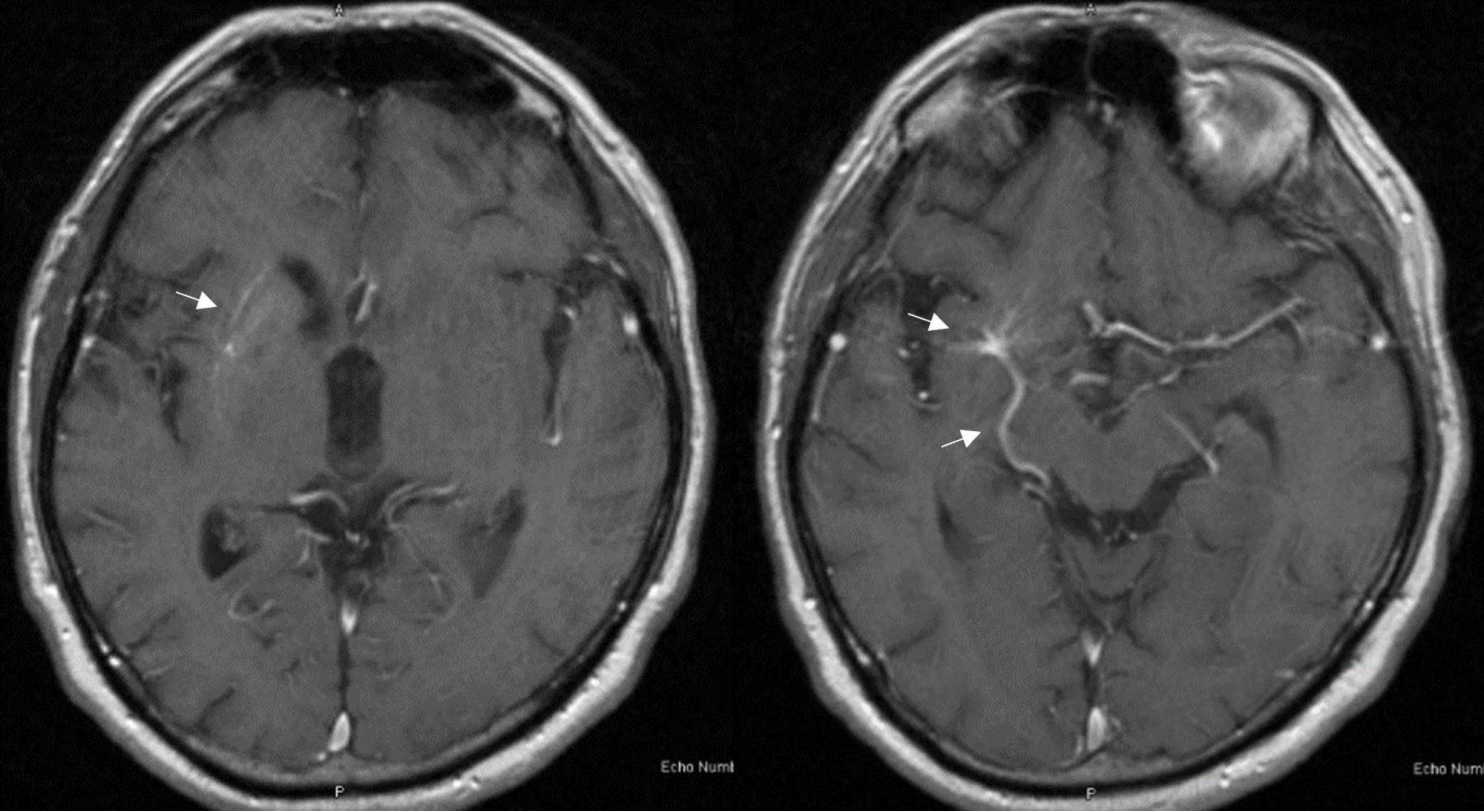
T1 magnetic resonance imaging (MRI) of the brain with contrast showing right-sided developmental venous anomaly (DVA)
